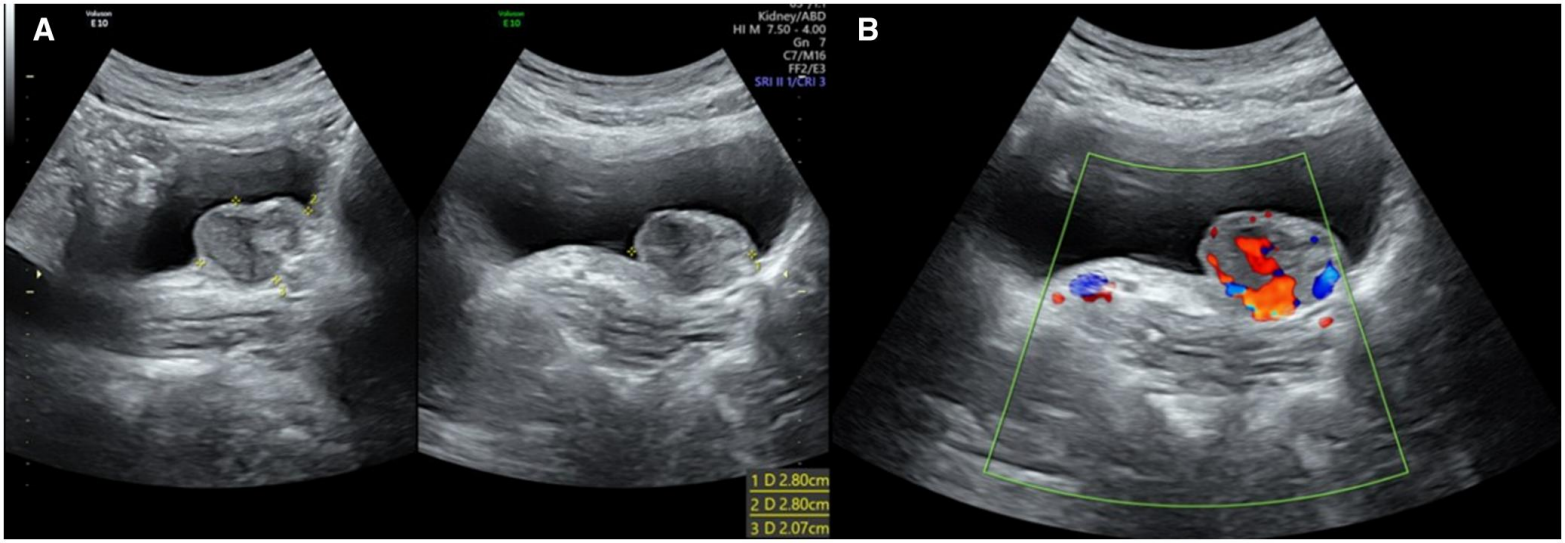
(A) Ultrasound image of the bladder showing the paraganglioma with corresponding size of 2.80 × 2.80 cm. (B) Colour doppler of the mass demonstrates increased vascularity of the lesion. Images were taken from the Gold Coast PACS system.
